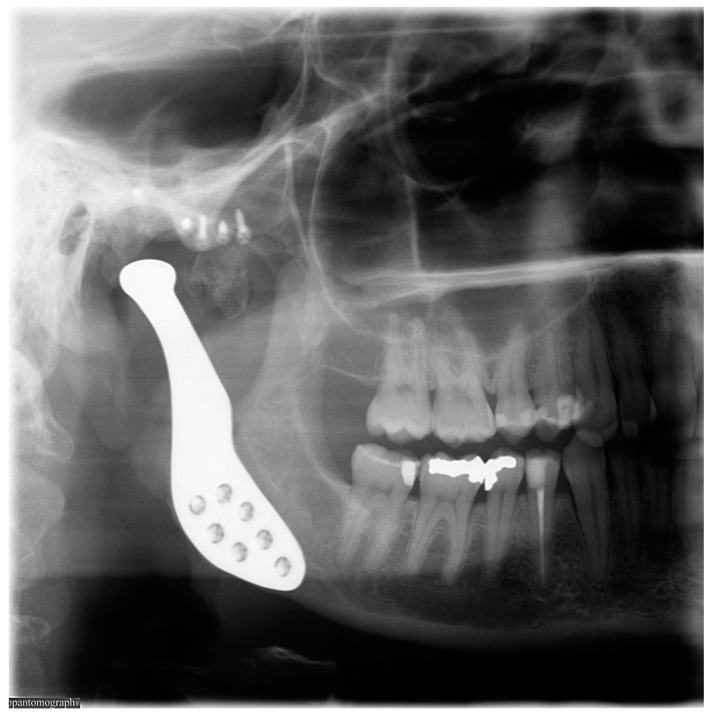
Postoperative orthopantomograph showing a custom total joint replacement.
